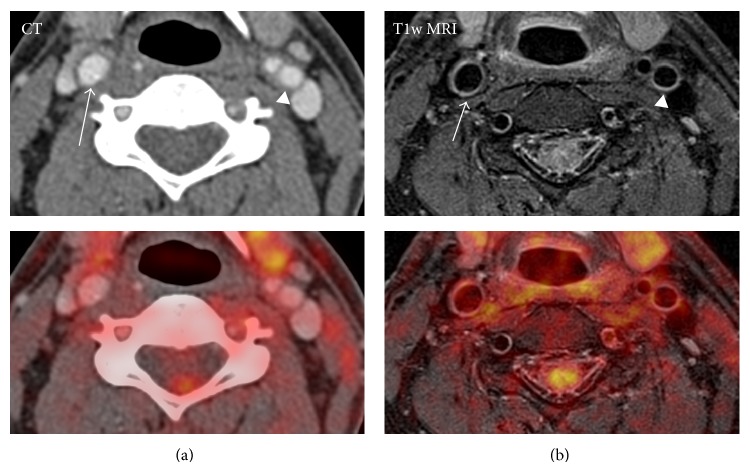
Comparison of contrast enhanced CT (a) with T1 weighted MRI (b) for vessel delineation in patients without significant carotid plaque. The right common carotid artery (arrow) and left internal carotid artery (arrowhead) are shown. The bottom row shows fusion with FDG-PET. This patient was part of a previous published trial [19].
